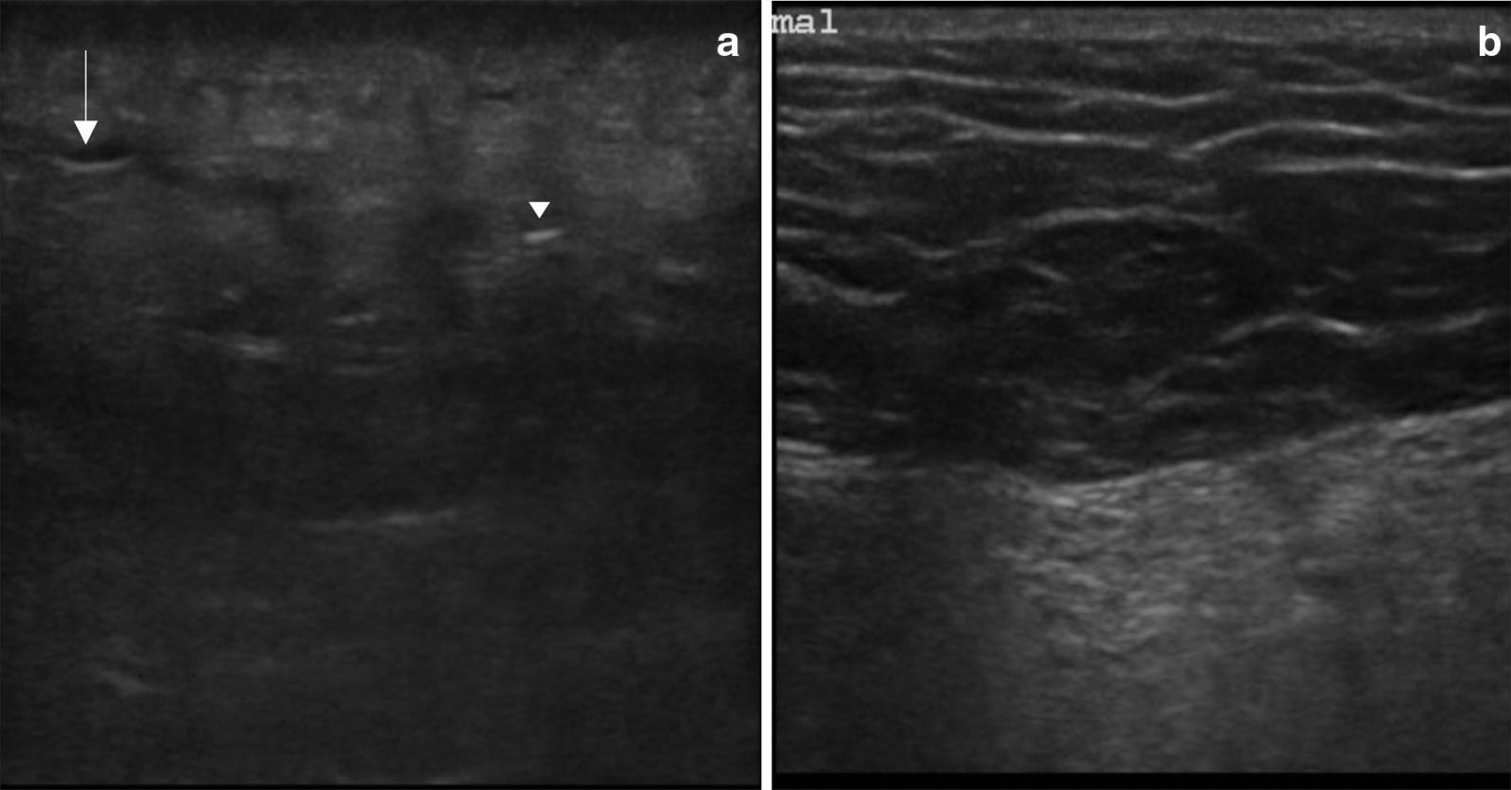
Point-of-care ultrasound scan of the affected left gluteal soft tissue (a) and normal soft tissue on the contralateral side (b) using a 15–6 MHz linear probe. In the affected tissue, there is loss of the normal subcutaneous architecture, diffuse increased echogenicity, hypoechoic regions that correspond to little fluid accumulations (arrow) and an hyperechoic focus with posterior dirty acoustic shadowing, corresponding to gas in the soft tissue (arrowhead)
